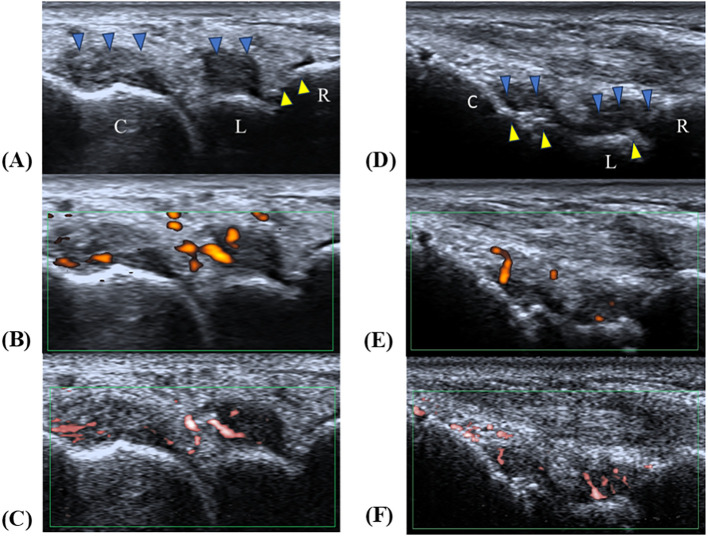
Case 1 (A–C): representative images of moderately active synovitis. (A) A longitudinal grayscale ultrasound scan of the radiocarpal joint shows grade III synovial hyperplasia (blue arrow) and grade I bone erosion (yellow arrow). (B) PDUS in the same plane reveals grade II blood−flow signals, indicating active inflammation. (C) SMI in the same plane also demonstrates grade II blood−flow signals, confirming active synovial neovascularization. Case 2 (D–F): Subclinical Synovitis in an RA Patient during Clinical Remission. (D) Longitudinal grayscale ultrasound of the radiocarpal joint demonstrates grade II synovial hyperplasia (blue arrow) and grade II bone erosion (yellow arrow). (E–F) In the same plane, PDUS shows grade I blood−flow signals, whereas SMI detects grade II signals. Both techniques confirm the presence of persistent subclinical synovial inflammation in this patient, with SMI exhibiting higher sensitivity than PDUS. R, radius; L, lunate; C, capitate; blue arrow, synovial hyperplasia; yellow arrow, bone erosion.
